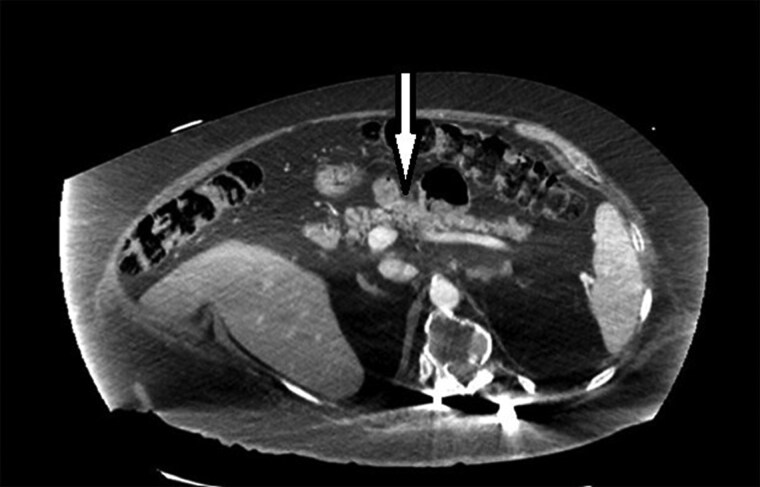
Computed tomography scan of abdomen with/without IV contrast shows fatty infiltration of the pancreas with moderate pancreatic atrophy although no evidence of hyper-enhancing focal masses to suggest insulinoma.
